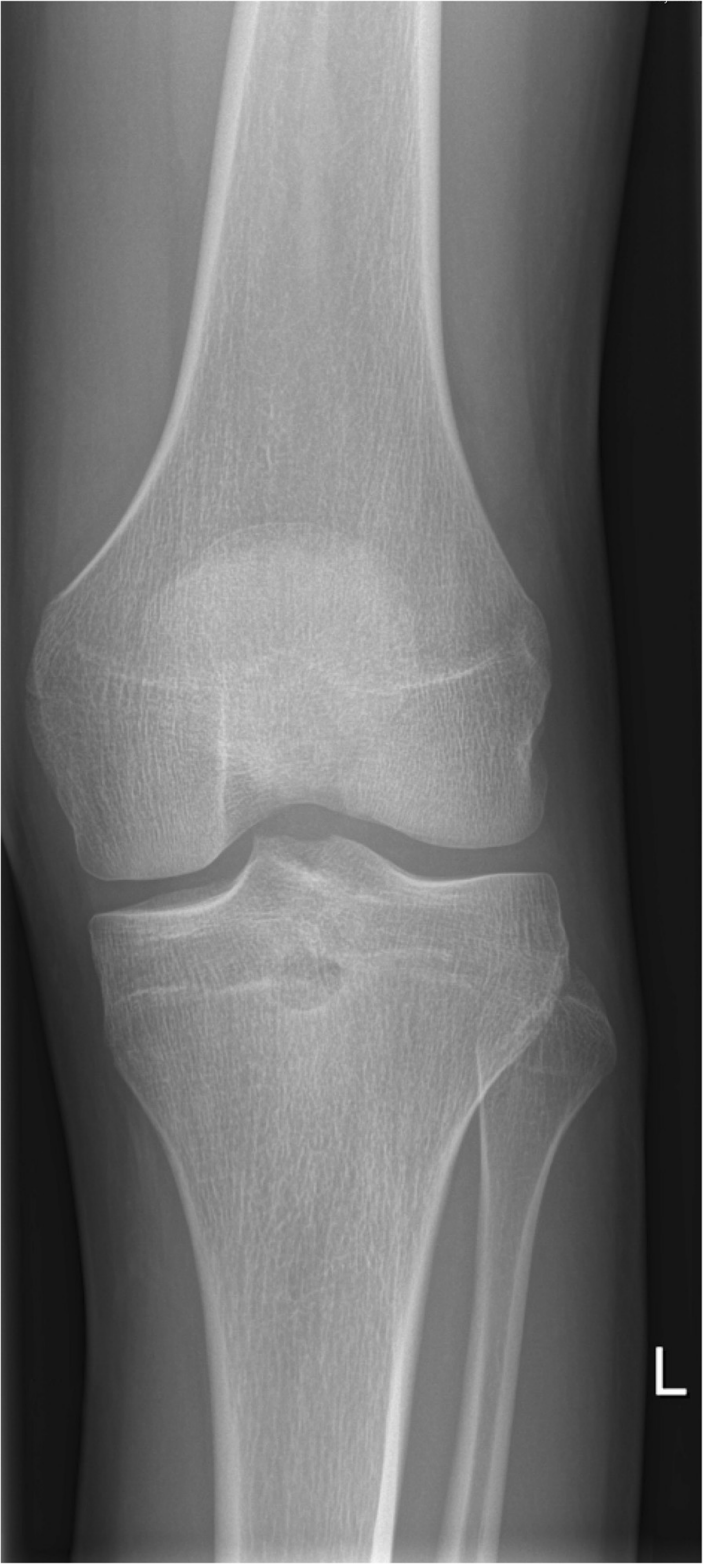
X-ray of the left knee (anteroposterior view), taken at the first postoperative out-patient presentation (10 weeks postoperative)
